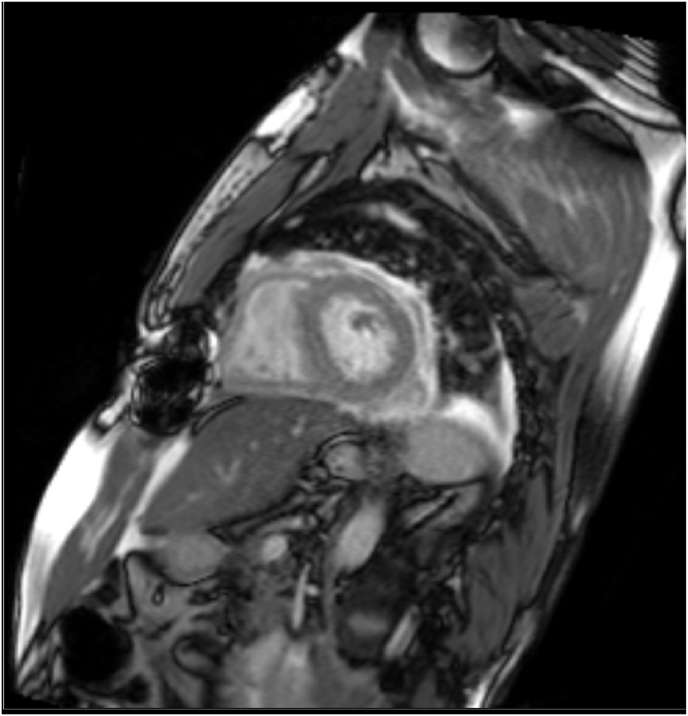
Cardiac MRI with small LV thrombus adhered to mid-to-distal inferior wall measuring 1.1x1.0x0.8cm.
